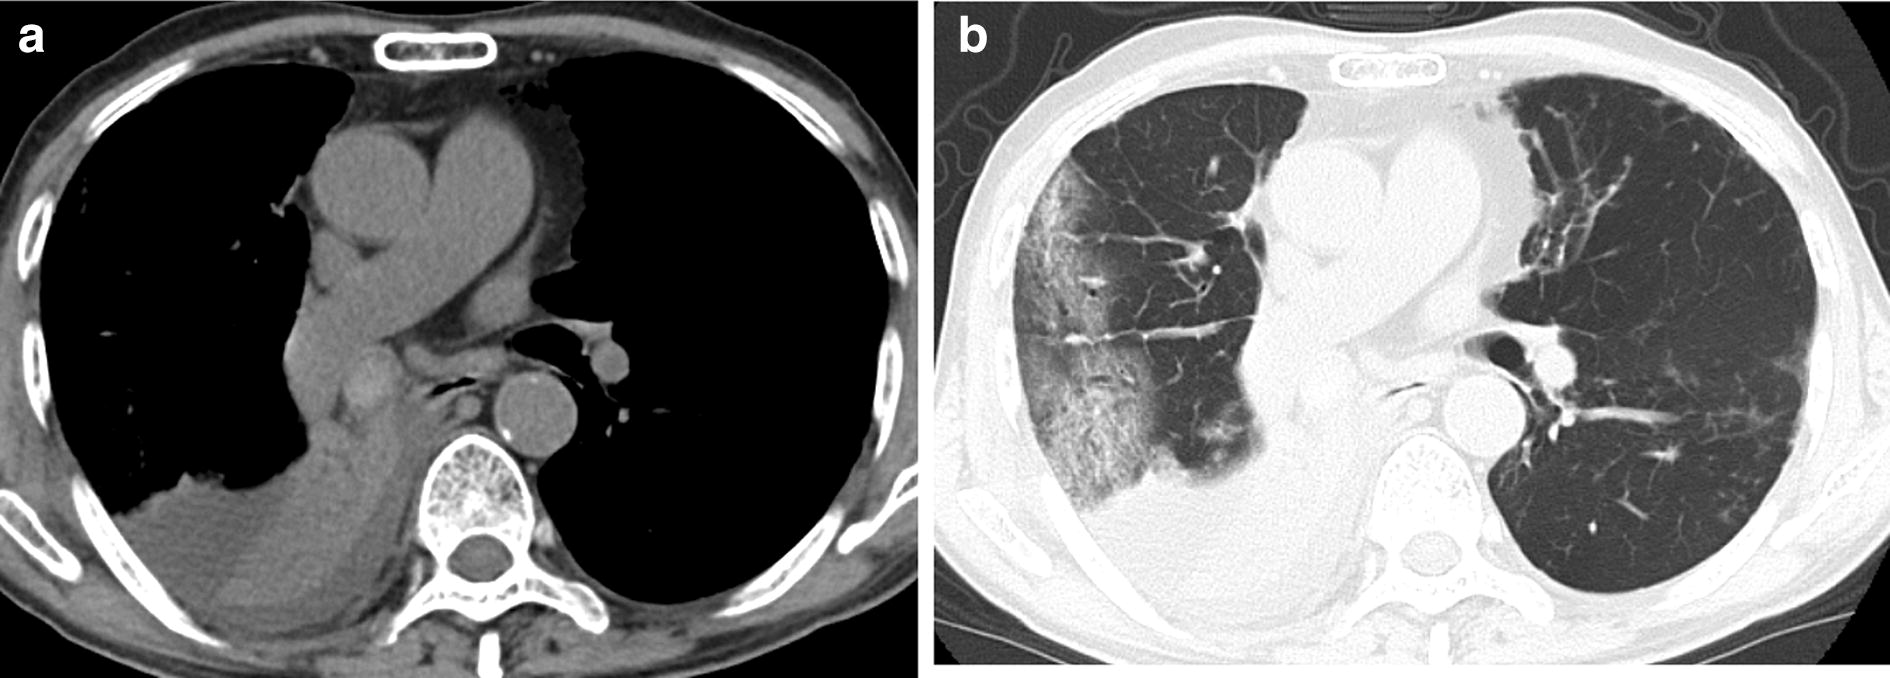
Computed tomography of chest. a High attenuation mucus plug in right intermediate bronchial trunk, and right pleural effusion. b Infiltration in right lung field
